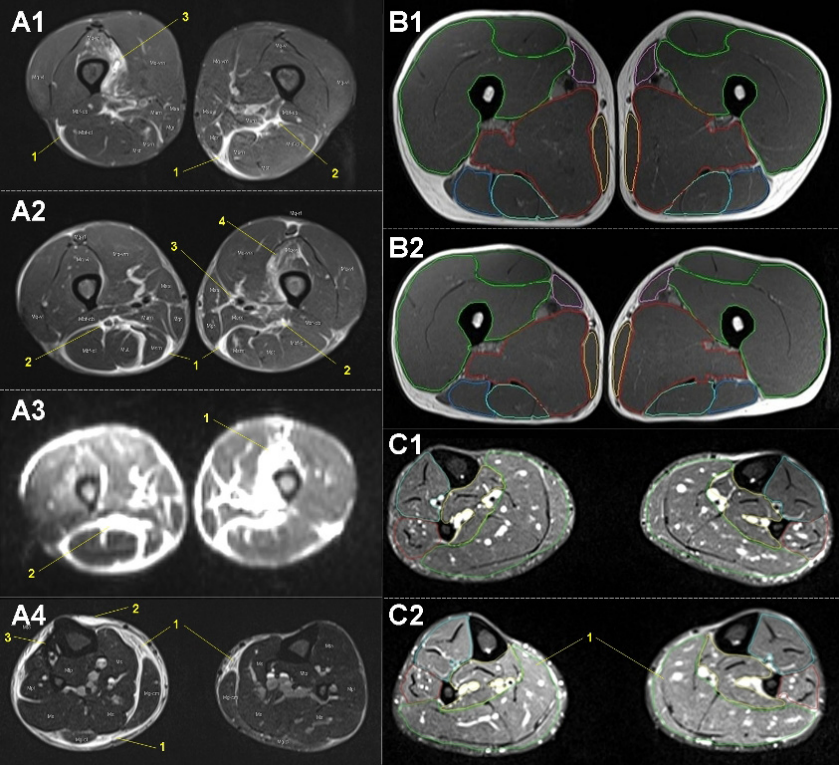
Mobile MRI of upper and lower legs. A: male, 49-years-old (coronal slices): A1: stage 12, 789 km, upper legs (PDw TSE fs): Subfascial intermuscular fluid, superficial (1), deep peri-neural (2). Partial quadriceps tear (M. vastus intermedius: 3). A2: stage 19, 1,260 km, upper legs (PDw TSE fs): Subfascial intermuscular fluid, superficial (1), deep peri-neural (2) and peri-vascular (3). Partial muscle edema of M. vastus intermedius (4). Specific diffusion weight imaging (A3, same slice as A2) is a sensitive method for free water detection. A4: stage 19, 1,260 km, lower legs (T2 TIRM): Subfascial intermuscular (1) and epifascial subcutaneous edema (2) indicating soft tissue inflammation such as perimyositis and panniculitis (shin splints), respectively. B: male, 31-years-old, B1: start, B2: stage 62, 6,358 km (PDw TSE fs transversal): Segmentation of muscle compartments of upper leg for functional muscle volumetry. C: male, 53-years-old: C1: start, C2: stage 46, 3,161 km (T2 TIRM transversal): Segmentation of muscle compartments of lower leg for functional muscle volumetry. Muscle edema in calf muscle (1).
